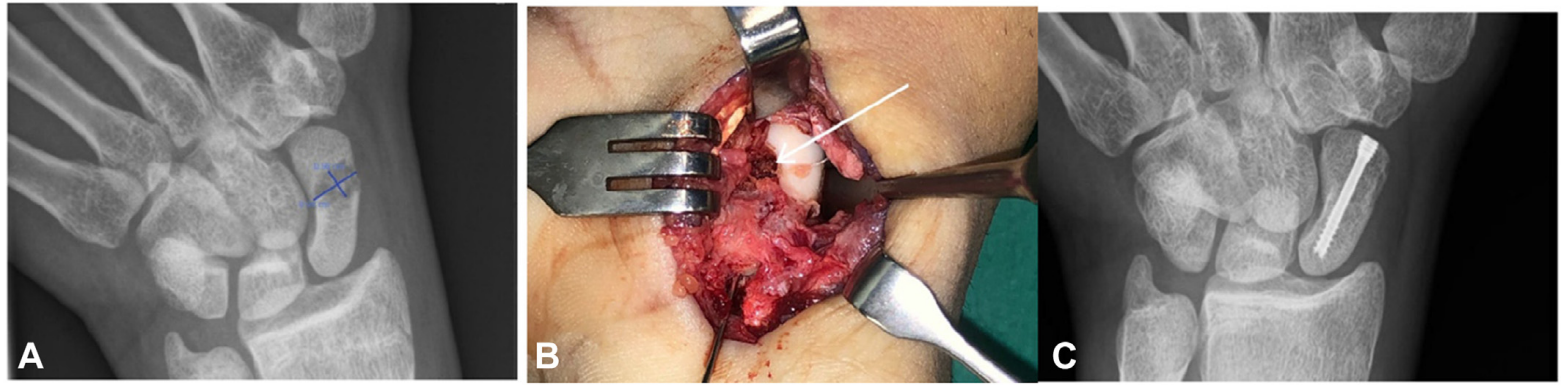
Case no 1. Distal pole fracture with palmar approach for “Perforate and Fill” bone grafting technique. (A) Radiograph showing the distal pole fracture with a large cavitation of L 9.4 mm × H 5.6 mm. (B) Surgical picture showing the fracture line is over the cartilage and fibrous part of the scaphoid, placement of the guide wire, and the white arrow showing the 4 mm burr hole access to the fracture cavity and bone grafts filling the cavity. (C) Radiograph showing sound union of the fracture.
